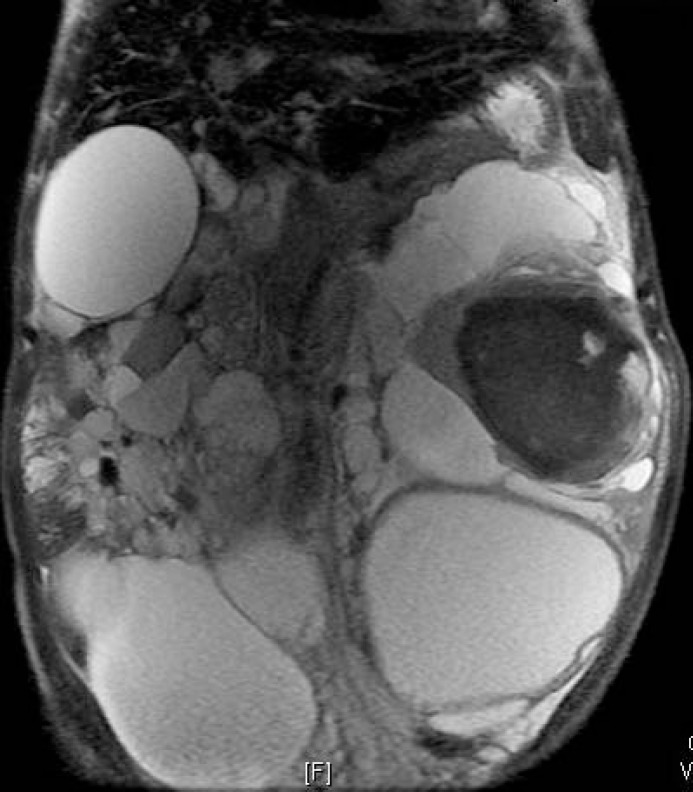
MRI showing bilateral polycystic kidneys with bleeding in left renal cyst.
